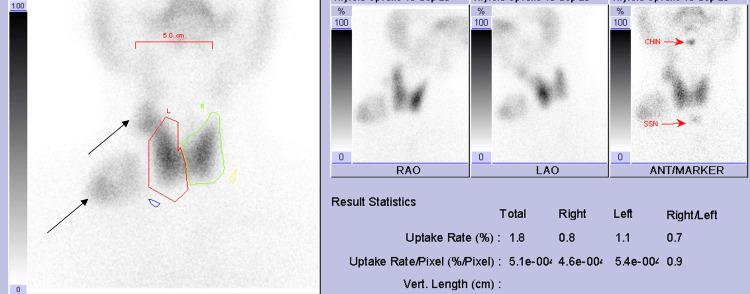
Planar images from thyroid uptake scintigraphy showing abnormal uptake in the right supraclavicular region and right mid-cervical region.
